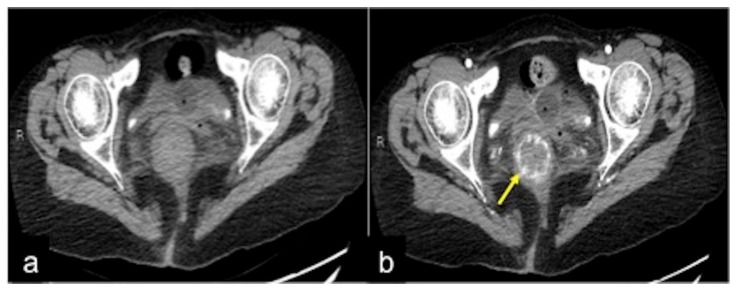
CTA multiphasic study of rectal bleeding during treatment with VEGF inhibitors. The pre-contrast (a) and arterial (b) phases show the presence of bleeding in the rectal wall (b, arrow) of a patient treated with Bevacizumab.
